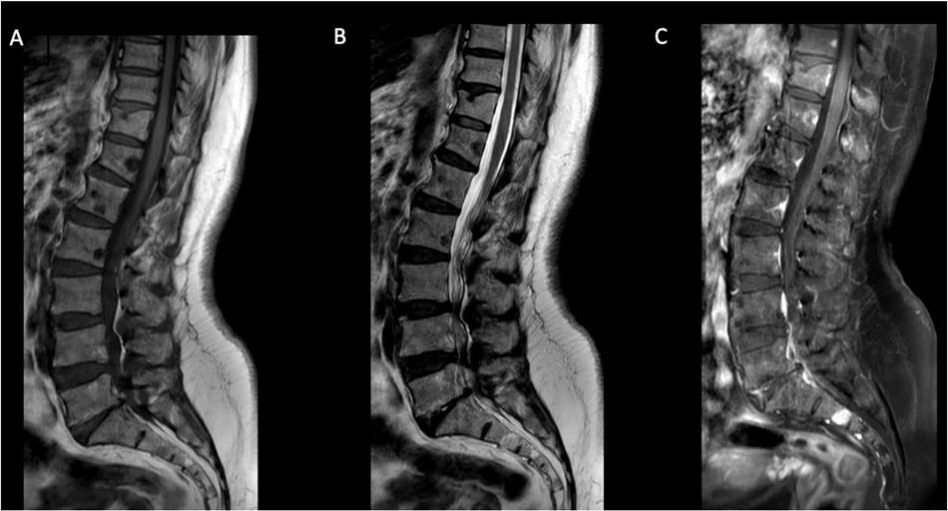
Spine MRI. Sagittal T1 (A) and T2 (B) reveal multiple hypo-intense vertebral metastases with enhancement (C).
